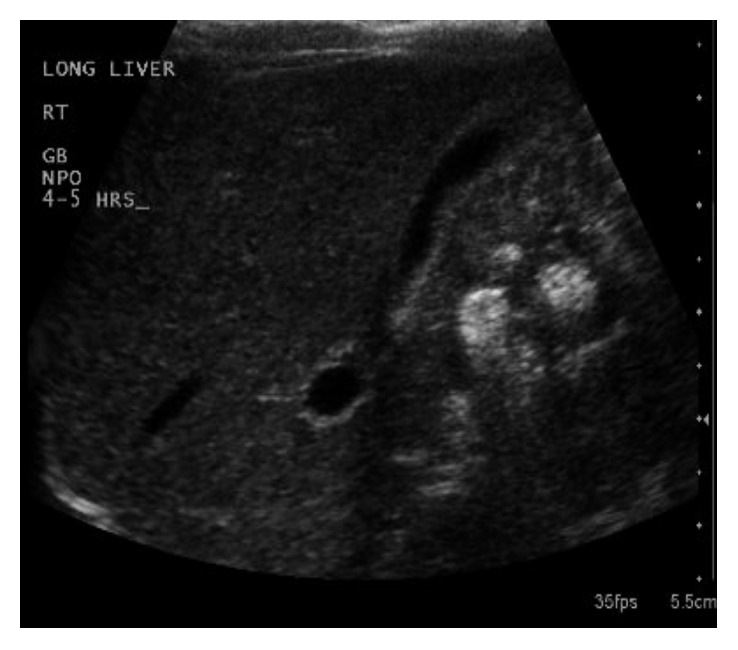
Abdominal ultrasound demonstrating a present gallbladder.
